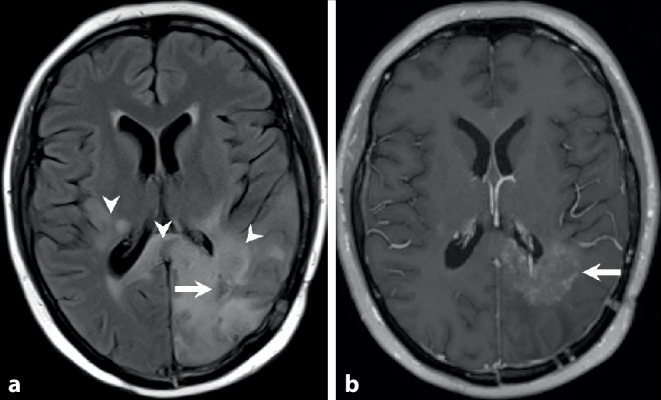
t = 14 weeks. Additional treatment with corticosteroids. The perifocal edema has clearly regressed on axial fluid attenuated inversion recovery (FLAIR) images (a, *arrow*). The signal intensity changes of the occipital forceps and the right sided perithalamic region have remained stable (a, *arrowheads*). On T1-weighted postcontrast images contrast (b) enhancement at the margins of the tumor resection has clearly regressed (*arrow*)
